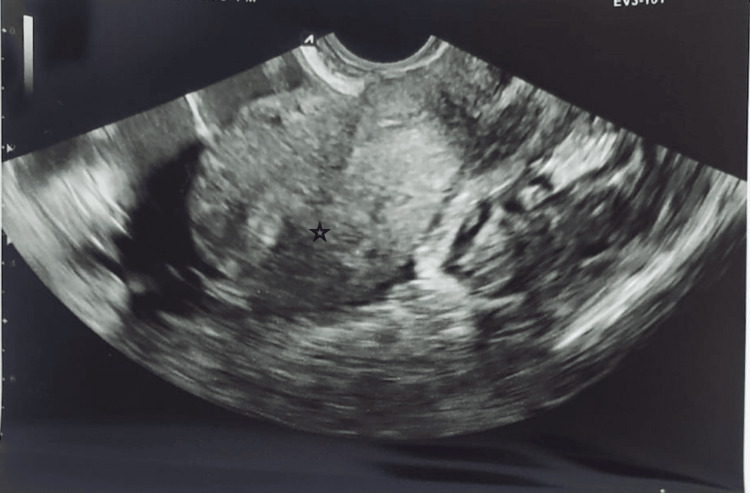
Ultrasonography shows an empty intrauterine sac (indicated by the star mark).
